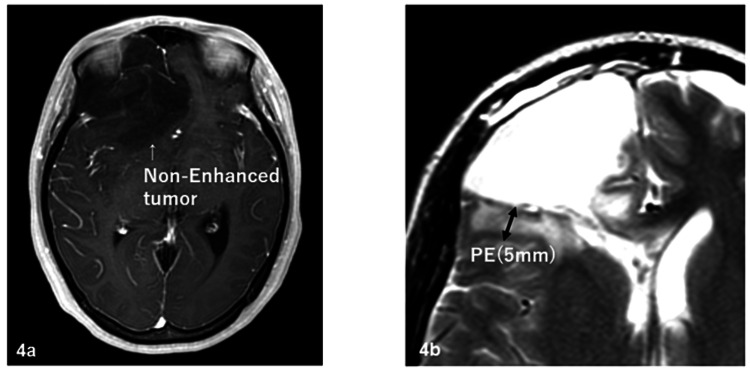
Supplementary MRI images – 4 4a: non-enhanced tumor before surgery; 4b: 5-mm PE after surgery MRI: magnetic resonance imaging; PE: preoperative edema
